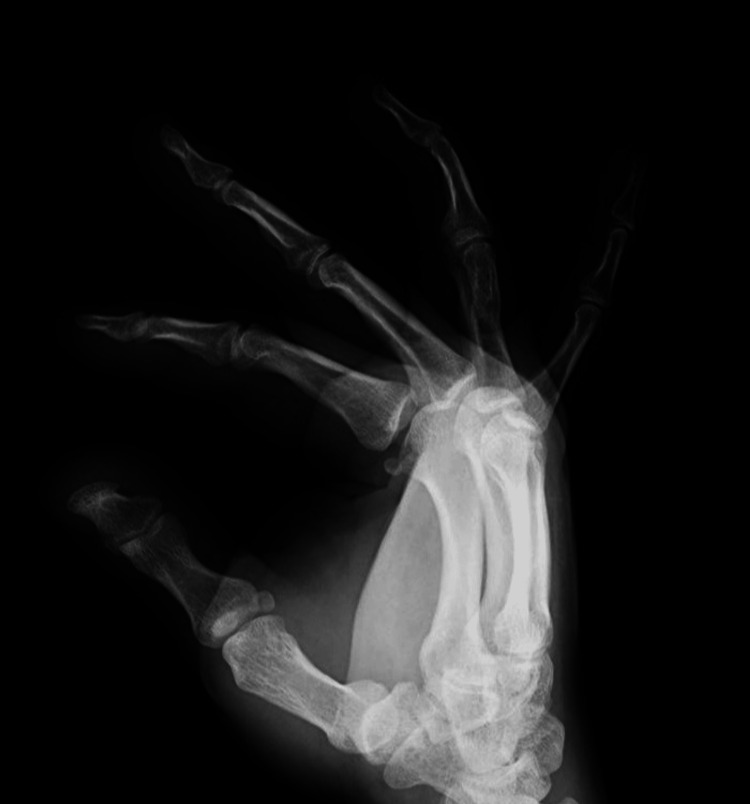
X-ray of the right hand in lateral projection, three years after the initial treatment with Kirschner wires
